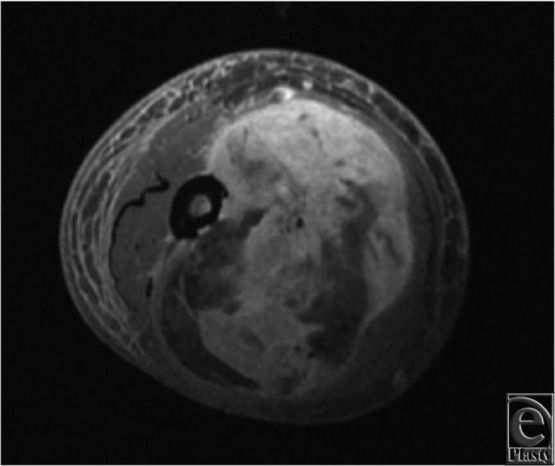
Magnetic resonance imaging of myxofibrosarcoma with invasion into the underlying humerus.
